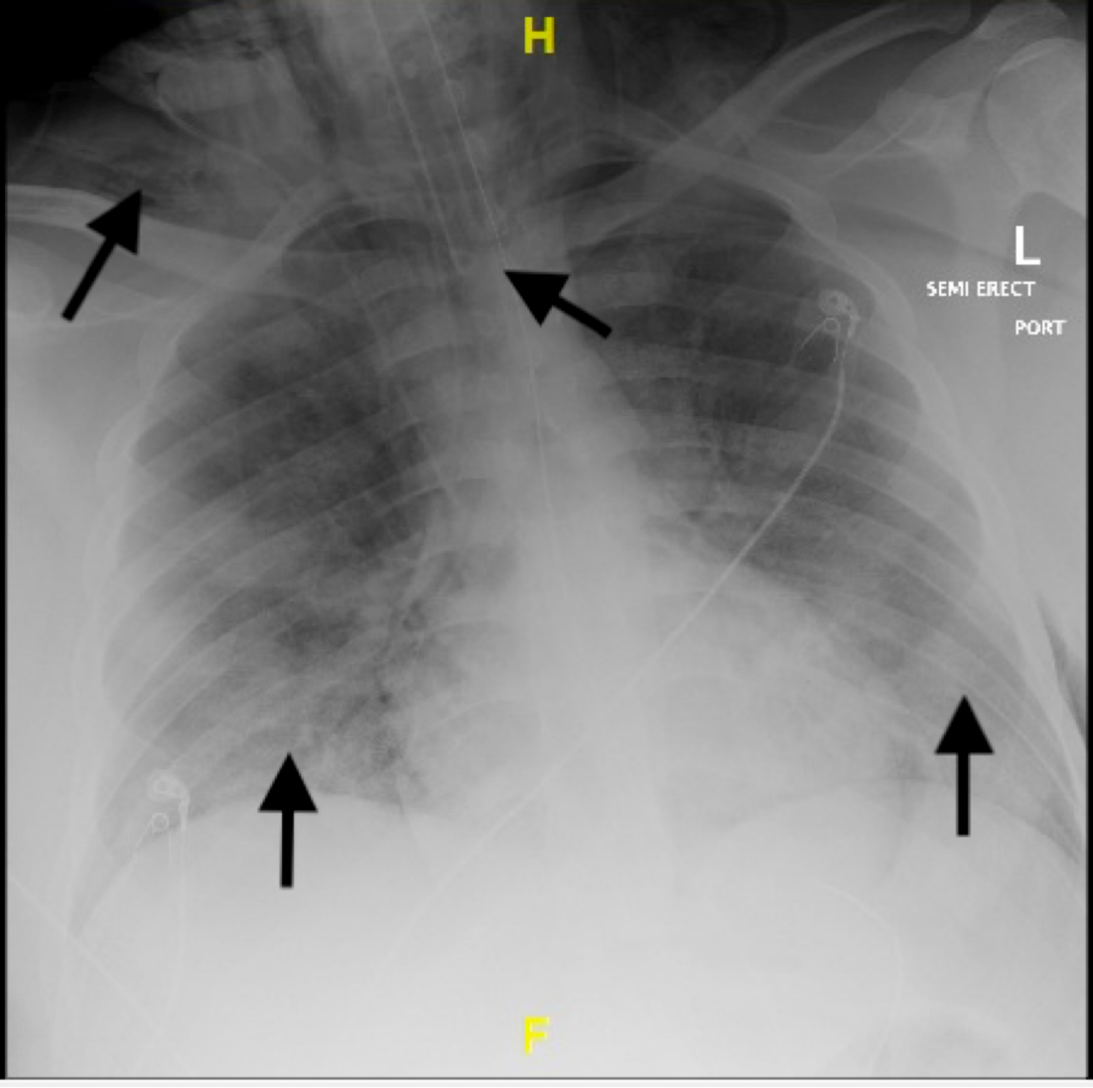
Post-intubation chest X-ray revealing endotracheal tube in appropriate position and slight worsening of the bilateral infiltrates along with subcutaneous emphysema.
